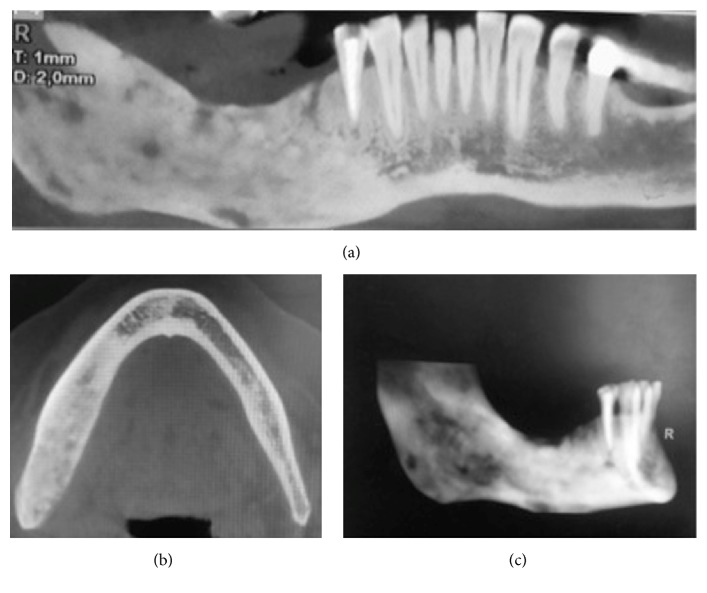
CT scan performed on April, 2015. (a) Panorex view showing sequestrum, (b) axial view, and (c) 3D reconstruction.
